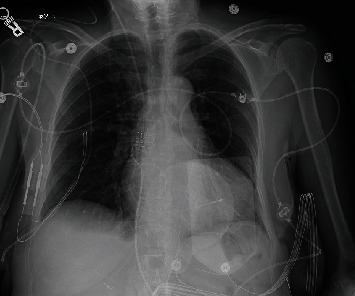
Chest X-ray single view with right jugular transvenous pacing wire with lead terminating in the right ventricle. There is a loop of the transvenous pacing wire seen within the right atrium.
